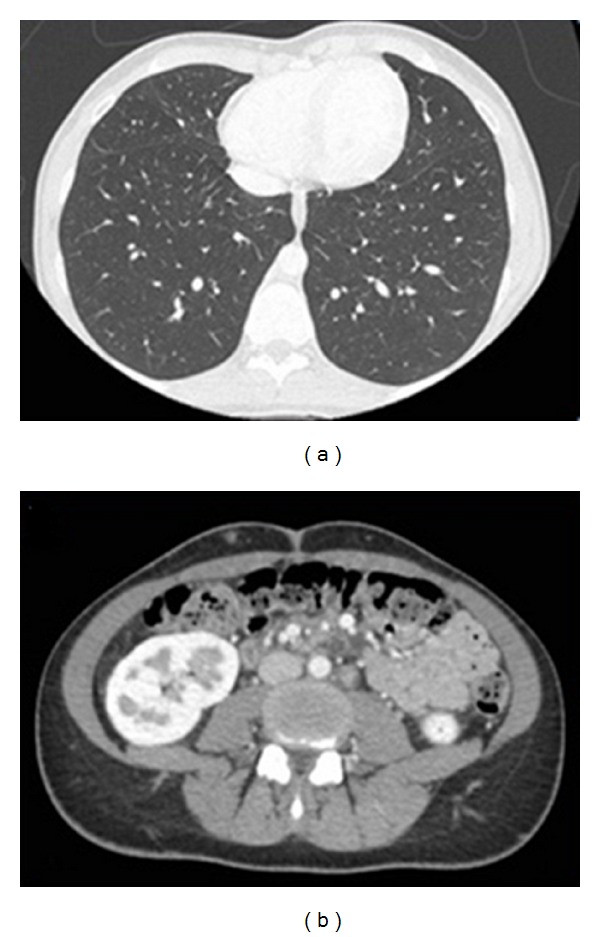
Computed tomography showing multiple pulmonary nodules (a) and retroperitoneal lymph nodes (b).
